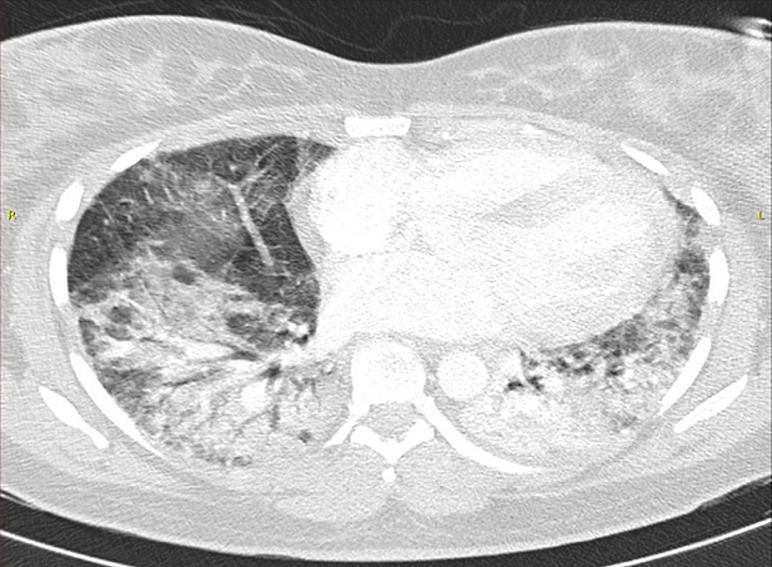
axial lung window CT demonstrating bilateral consolidation in bilateral posterior basal lower lobes
